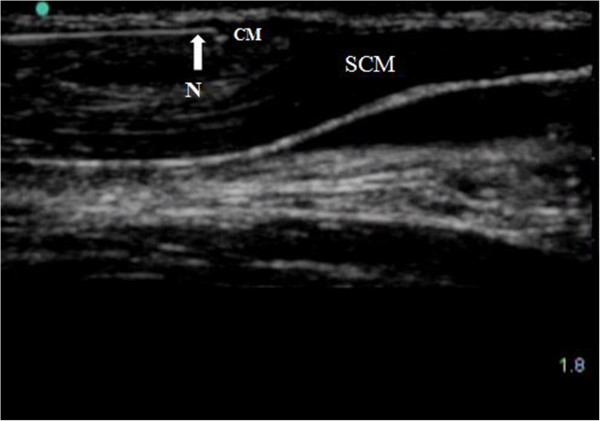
The ultrasound-guided anterior cervical cutaneous nerve block. N, needle; CM, contrast medium; SCM, sternocleidomastoid muscle.
